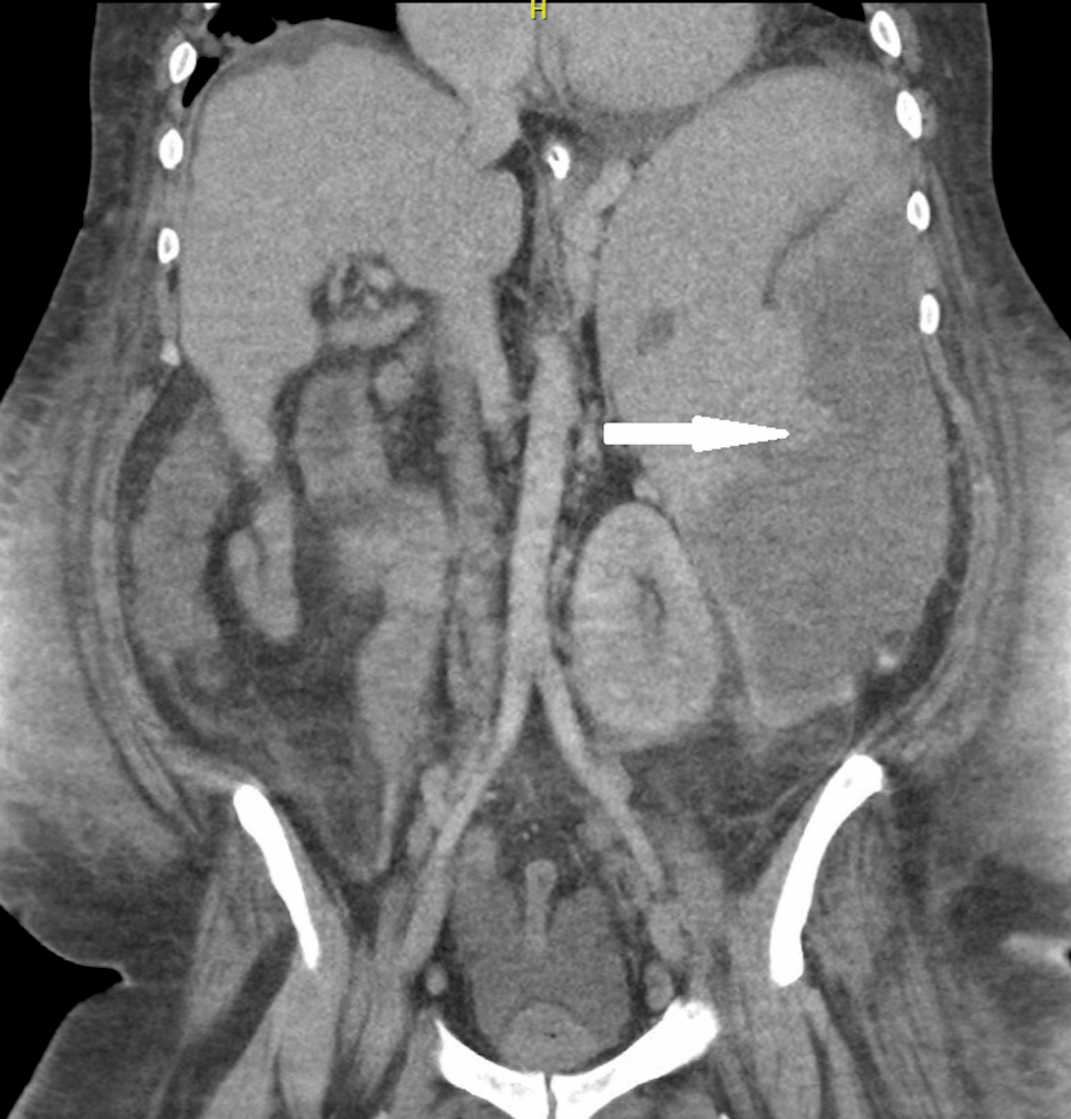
CT abdomen showing splenic infarct (white arrow)
